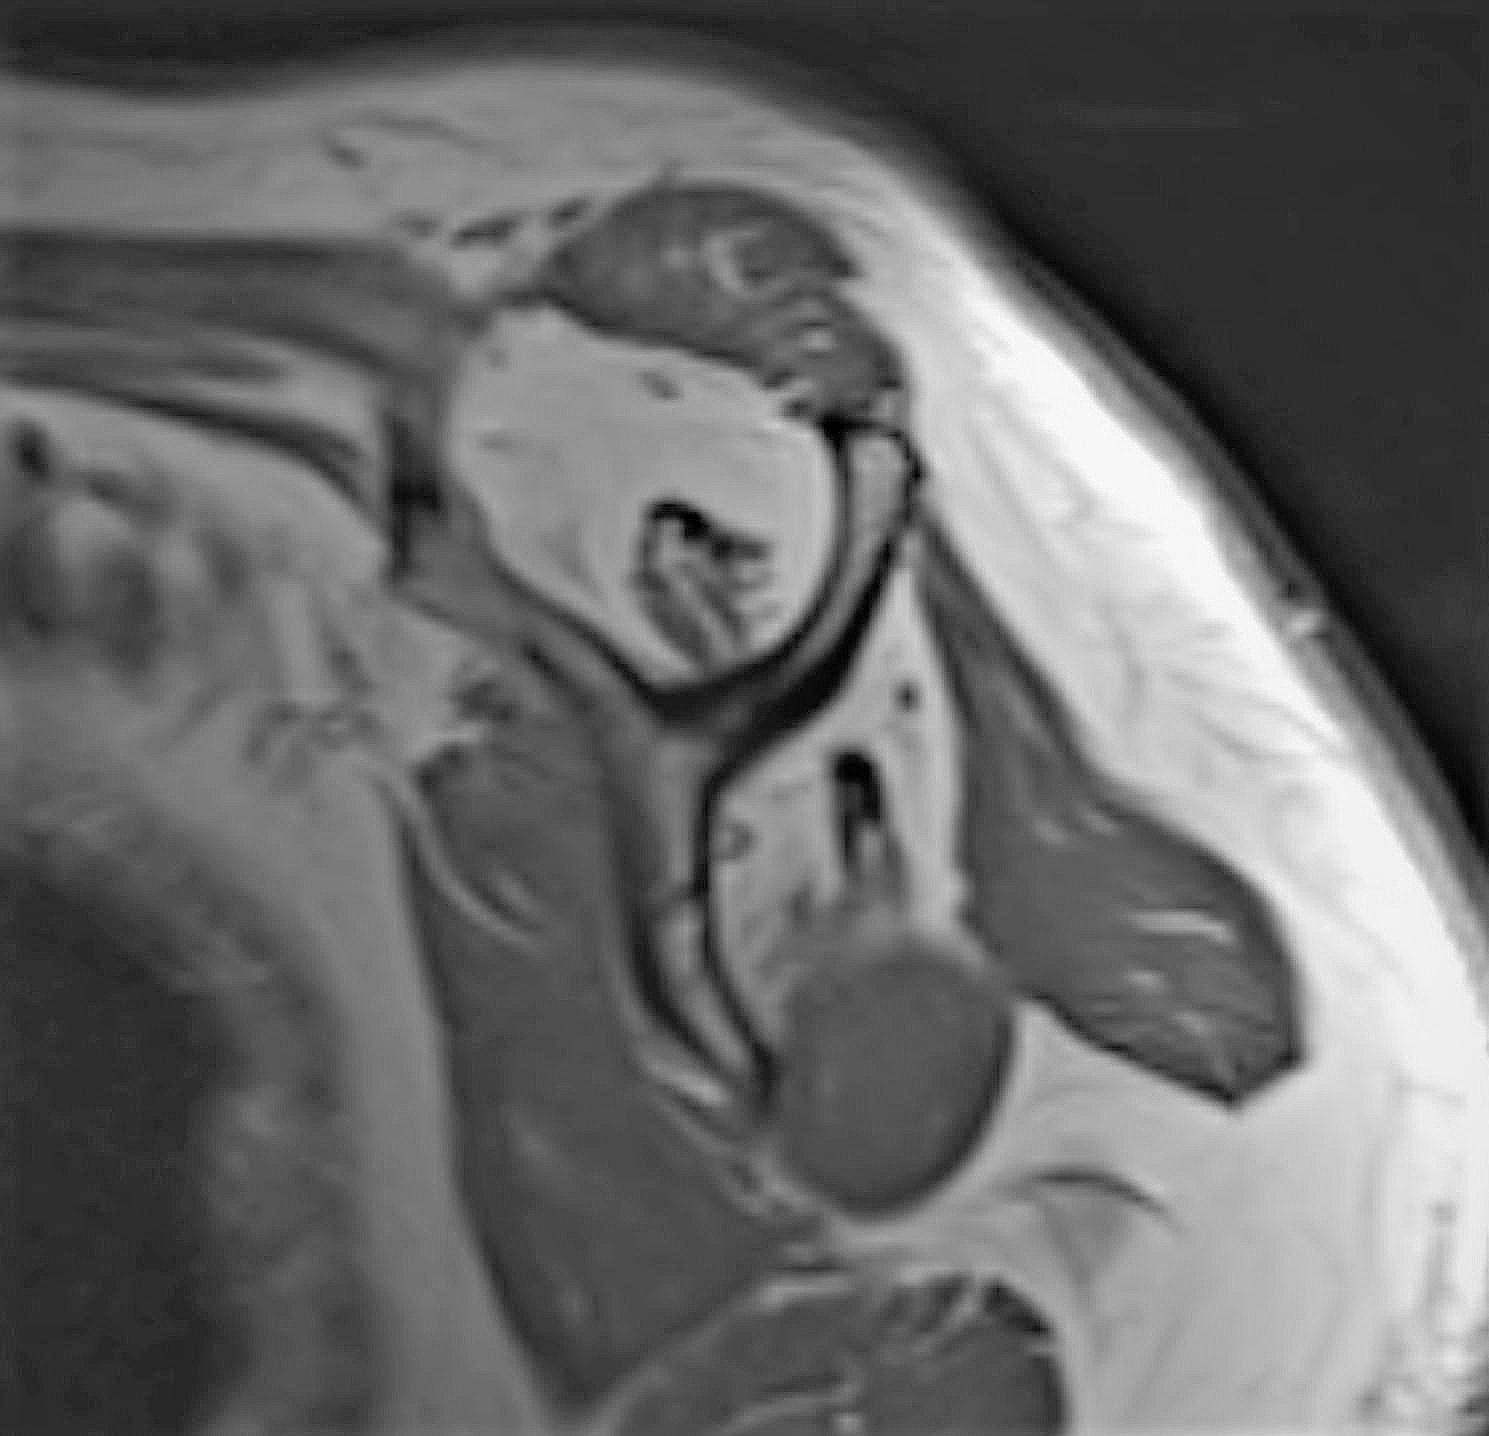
Images of a case sample of Hamada grade 3, the most common type detected in the study. 2a: T1-weighted oblique sagittal MRI section
